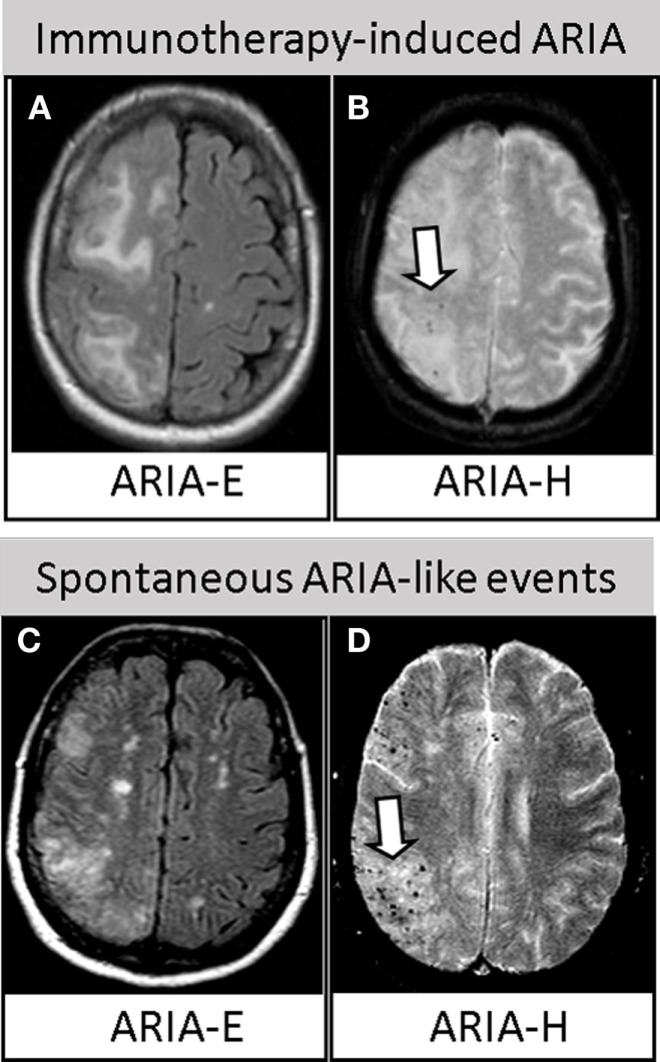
Similarities between immunotherapy-induced ARIA and spontaneous ARIA-like events in CAA-ri. *Upper row*. Axial brain MRI revealing ARIA-E (A) and ARIA-H [(B), arrow] in one AD patient treated with bapineuzumab. Reproduced with permission from Ref. (3). *Lower row*. Axial brain MRI revealing spontaneous ARIA-E (C) and ARIA-H [(D), arrow] occurring in one CAA-ri patient from the “The inflammatory Cerebral Amyloid Angiopathy and Alzheimer’s disease βiomarkers (*i*CAβ) International Network (20, 50).” Images (A,C) represent FLAIR-MRI sequences; (B,D) represent T2*-GRE sequences.
